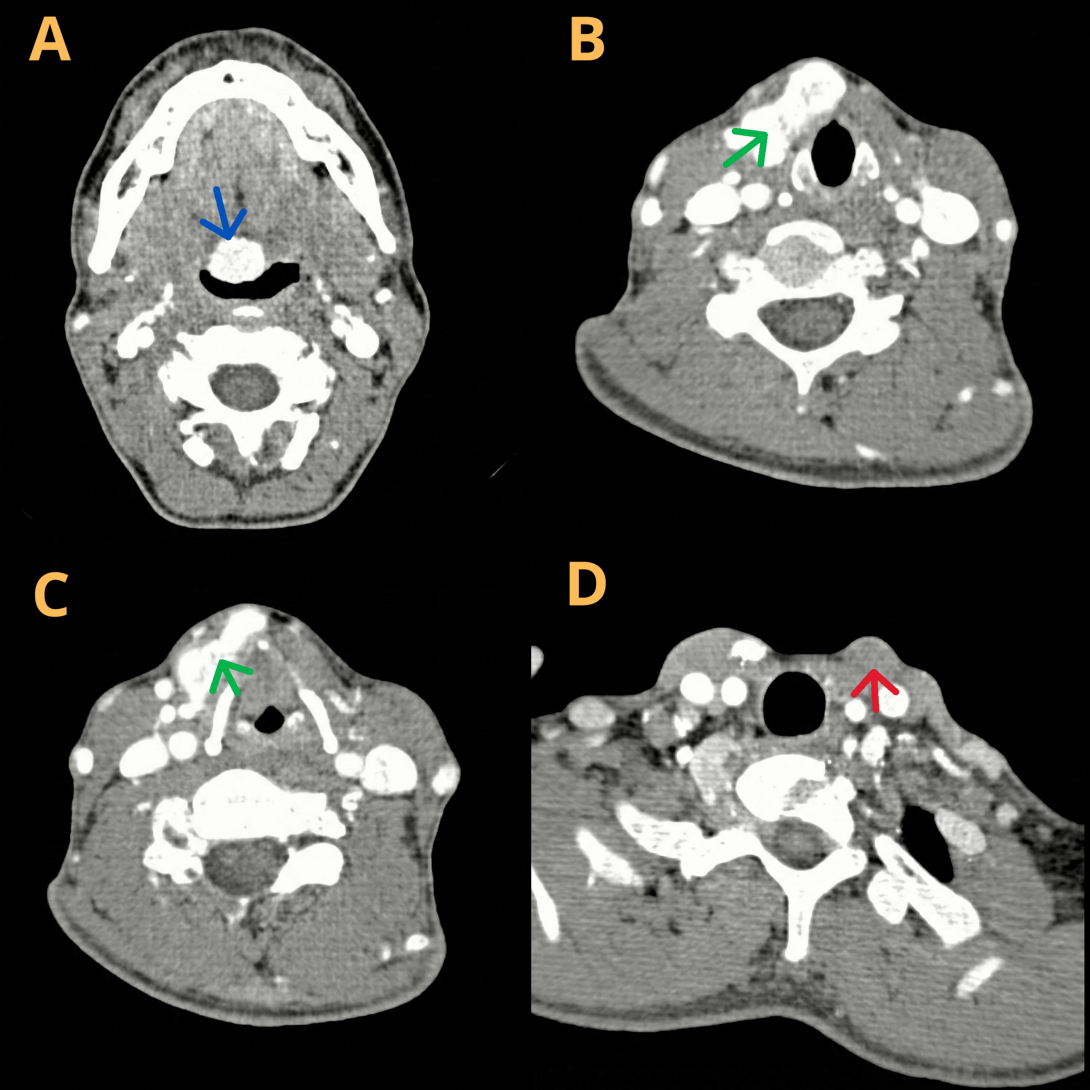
Sagittal computed tomography of the cervical region. Hypervascular expansile lesions at the base of the tongue (A) and in the visceral neck area at the level of the larynx (B, C) and absence of an orthotopic thyroid gland (D). Red arrow: empty thyroid cavity; Green arrow: thyroid parenchyma adjacent to the larynx; Blue arrow: thyroid at the base of the tongue.
